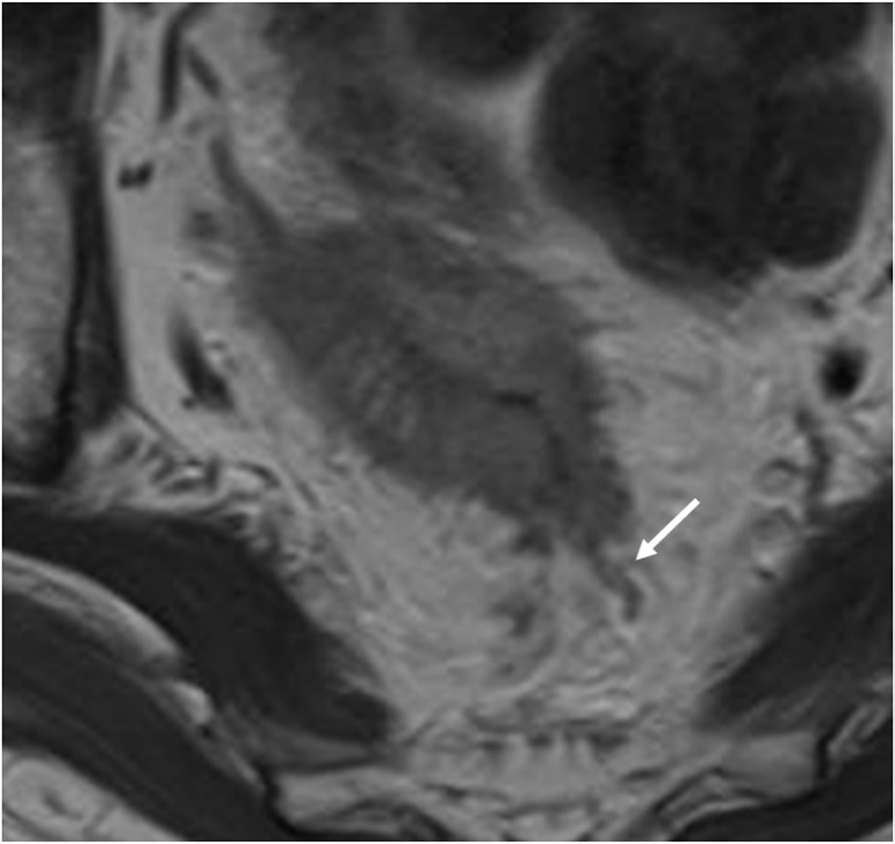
Extramural venous invasion detected with magnetic resonance imaging. Tumor signal intensity spread beyond the rectal wall. And irregular vessel contour or nodular expansion of vessel with definite tumor signal is demonstrated. MRI-EMVI score 4 (white arrow)
